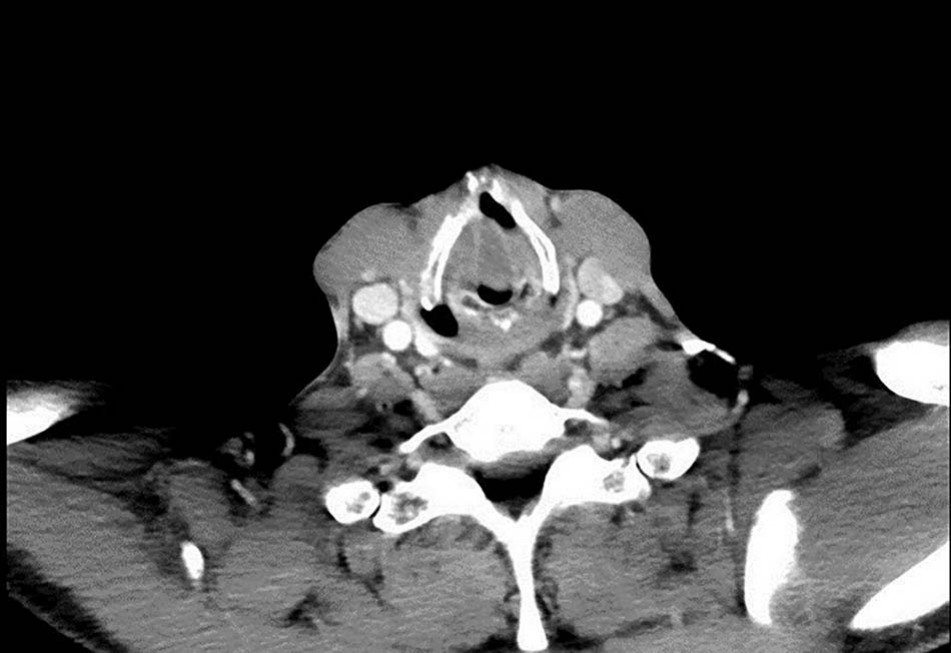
Axial CT showing obstruction at the level of the vocal cords. Media: video showing the laryngocoele.
